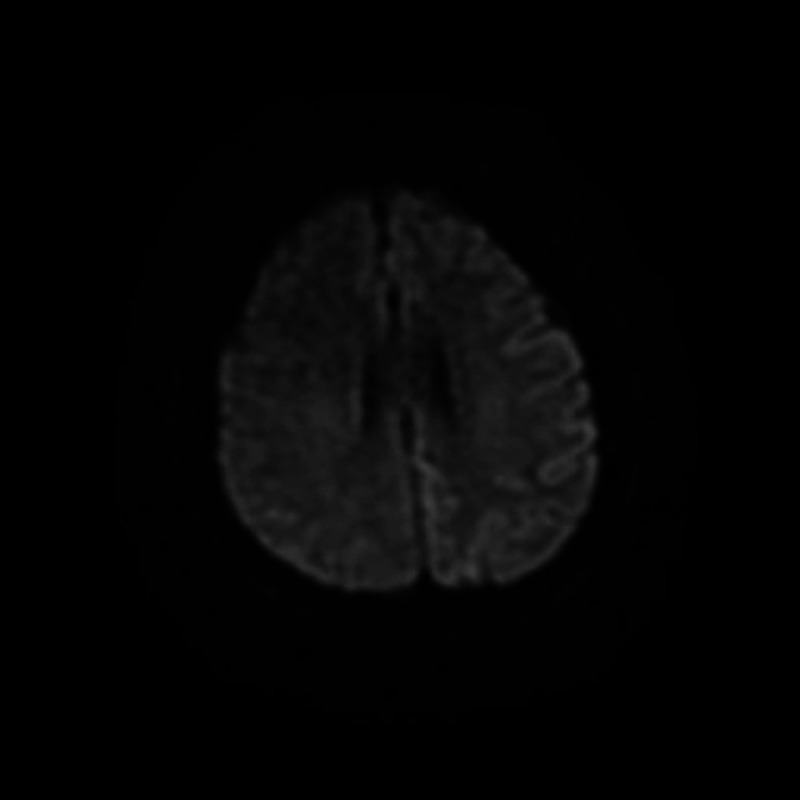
MRI DWI image showing bright signals in various areas of MR cortex consistent with cortical ribboning MRI: magnetic resonance imaging; DWI: diffusion-weighted imaging
